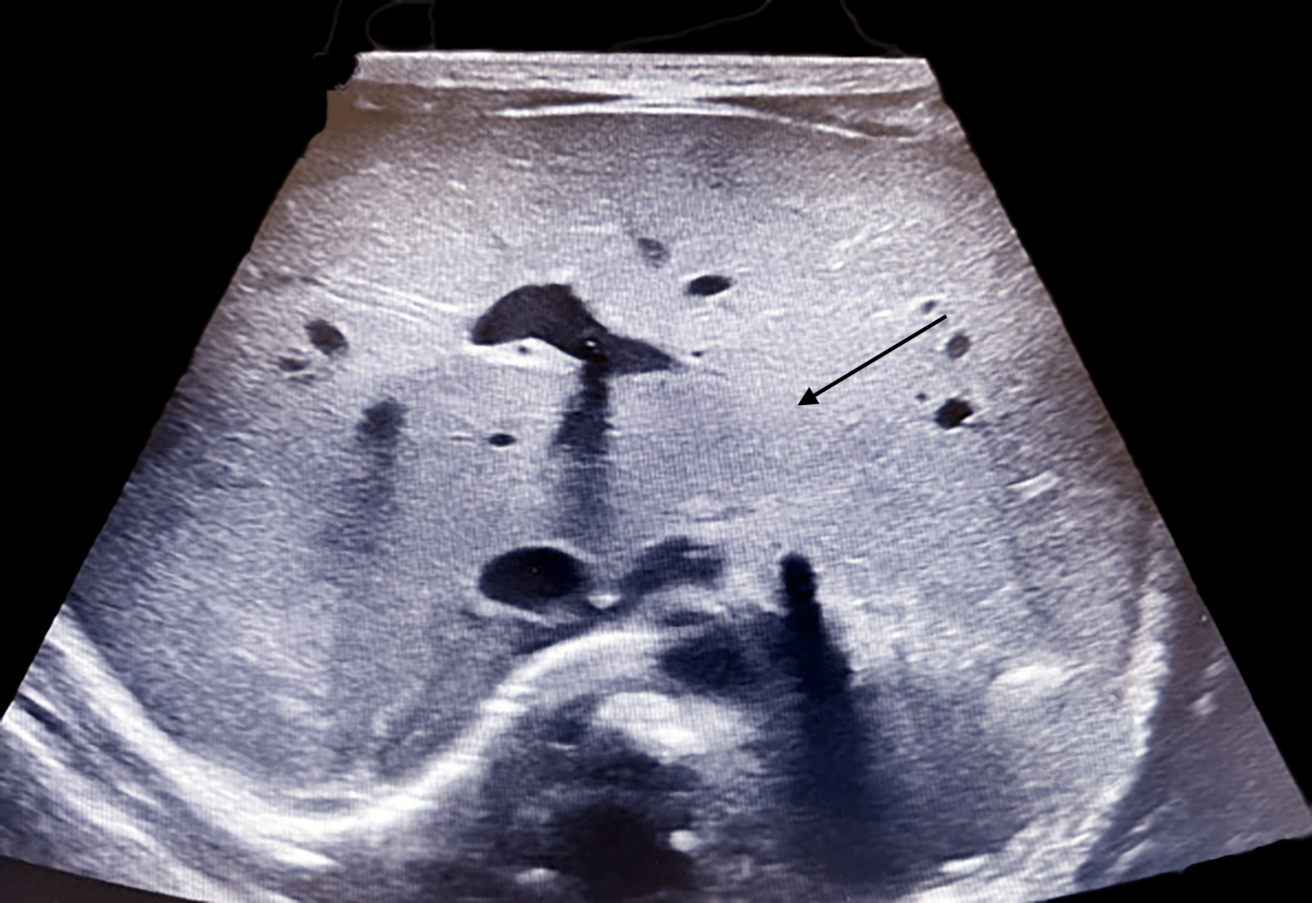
Transverse ultrasound view of the upper abdomen showing a liver extending across the midline (black arrow)
